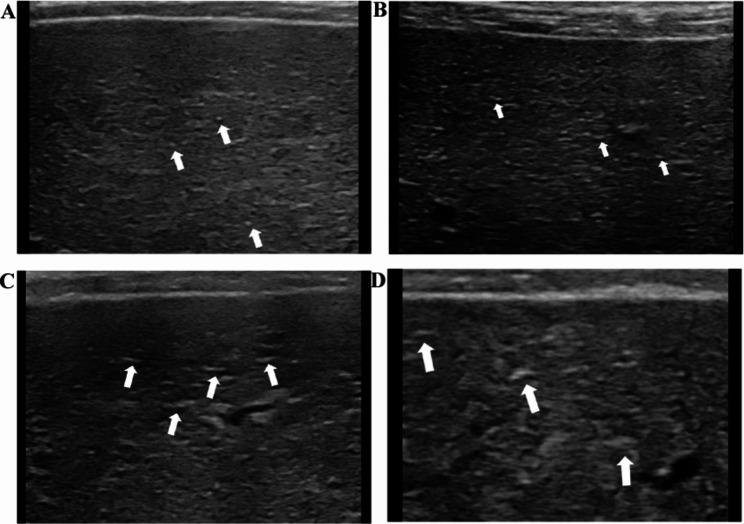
The ultrasound findings of the liver echo texture. (A) Fine (≤ 1 mm); (B) mildly coarse (2-5 mm); (C) coarse (> 5 mm); (D) small nodules (≤ 3 mm)
